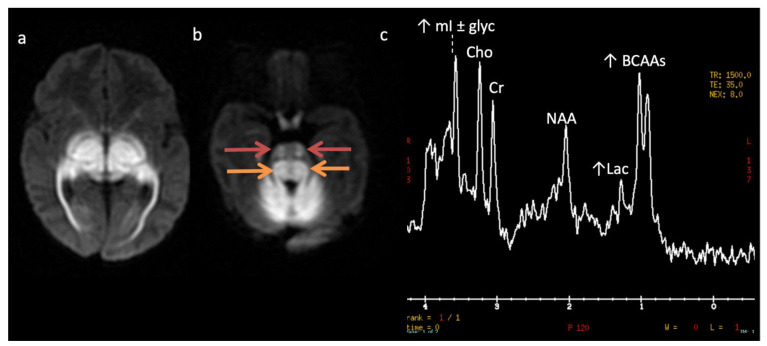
Maple syrup urine disease (MSUD) in a 23-day-old male. (a,b) Axial DWI through the level of the deep cerebrum (a) and pons (b) demonstrate diffuse symmetric markedly reduced diffusion in keeping with intramyelinic edema in the myelinated white matter tracts. Involved regions include the globi pallidi, internal capsules, thalami, and optic radiations (a) and the cerebellum, brainstem tracts, and optic chiasm (b). Note the appearance of the brainstem with involvement of the bilateral corticospinal tracts (ventral red arrows, b) and central tegmental tracts (dorsal orange arrows, b). (c) SV-MRS over the left basal ganglia (TR 1500, TE 35 ms) reveals a large broad doublet peak at 0.9 ppm consistent with branched chain amino- and keto-acids (BCAAs, BCKAs) and Lac. Additional findings include elevated mI and/or glycine at 3.6 ppm and mildly reduced NAA and Cho.
